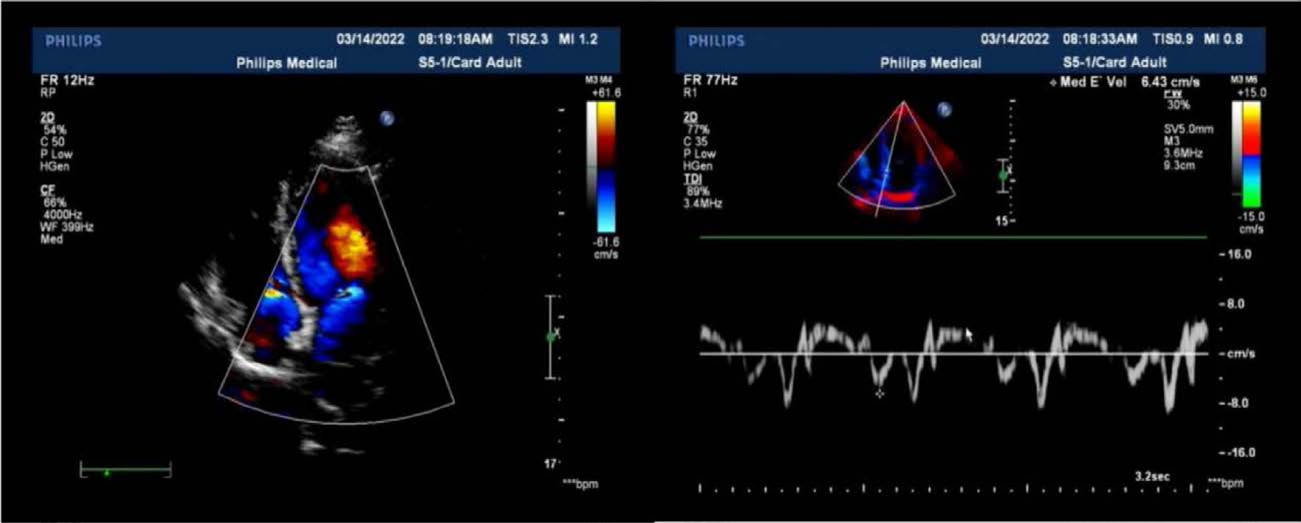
Transthoracic echocardiography after resolution of TC. No obvious abnormality of wall motion is visible. Aortic valve regurgitation was minimal. TC: Takotsubo cardiomyopathy.
